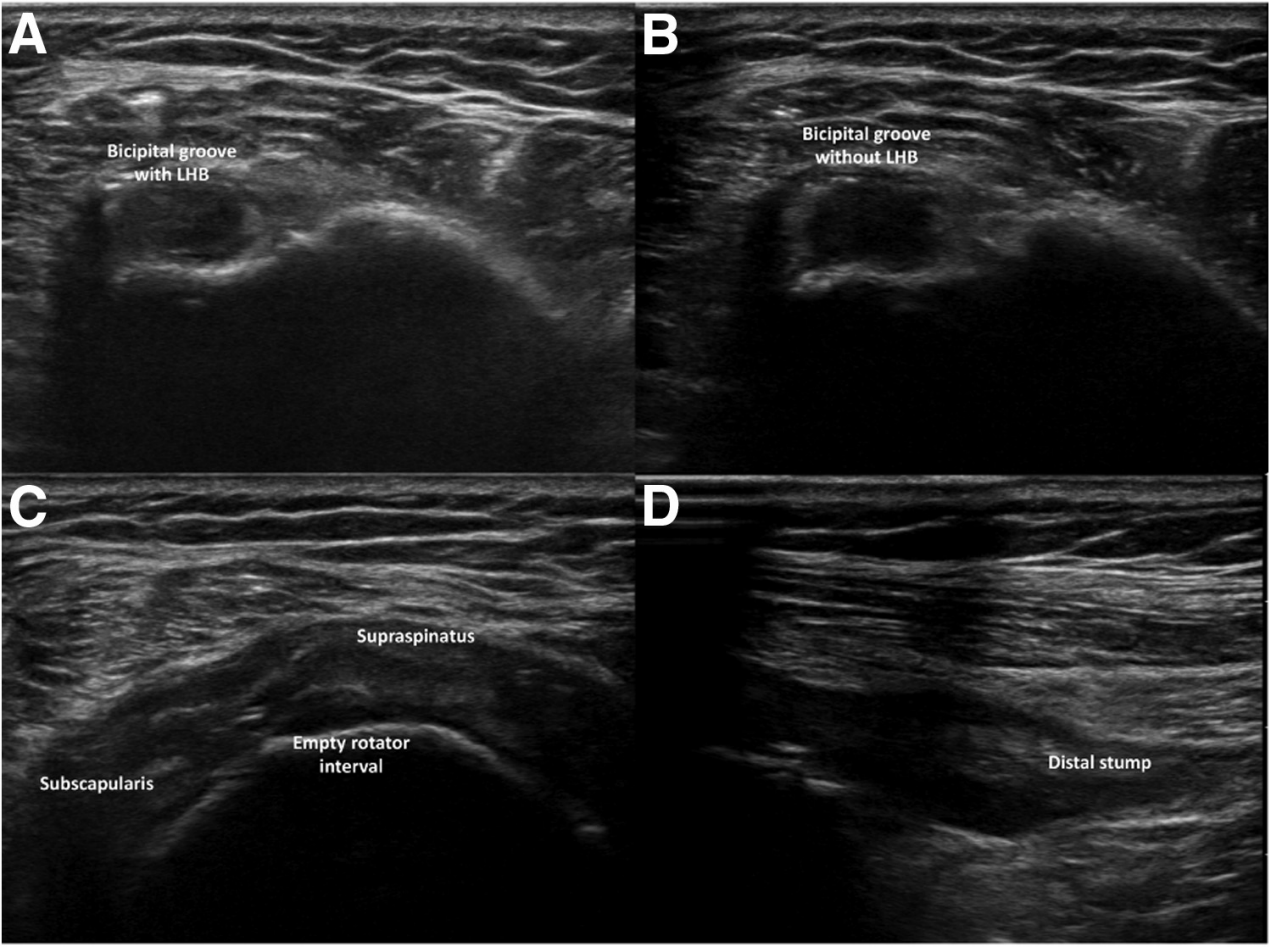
Ultrasound check before (A) and after in-office biceps tenotomy: the groove is empty (B, C) and the biceps stump is found more distally (D). (LHB, long head of the biceps.)
